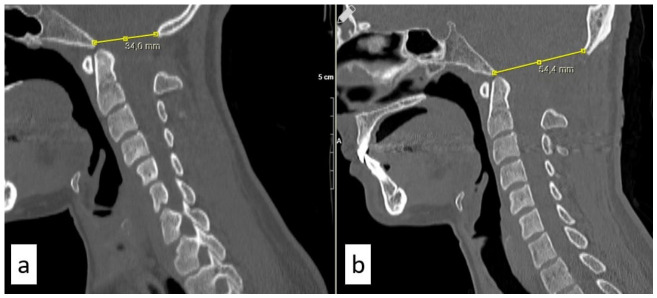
Representative sagittal CT images demonstrating the measurement of the anteroposterior diameter of the foramen magnum at the McRae line. (a) Pre-operative image showing the foramen magnum diameter, and (b) post-operative image demonstrating the new foramen magnum diameter following suboccipital decompression, measured in millimeters. Both images are from the same patient. The McRae line is defined between the basion and opisthion, and the anteroposterior diameter was measured along this line.
